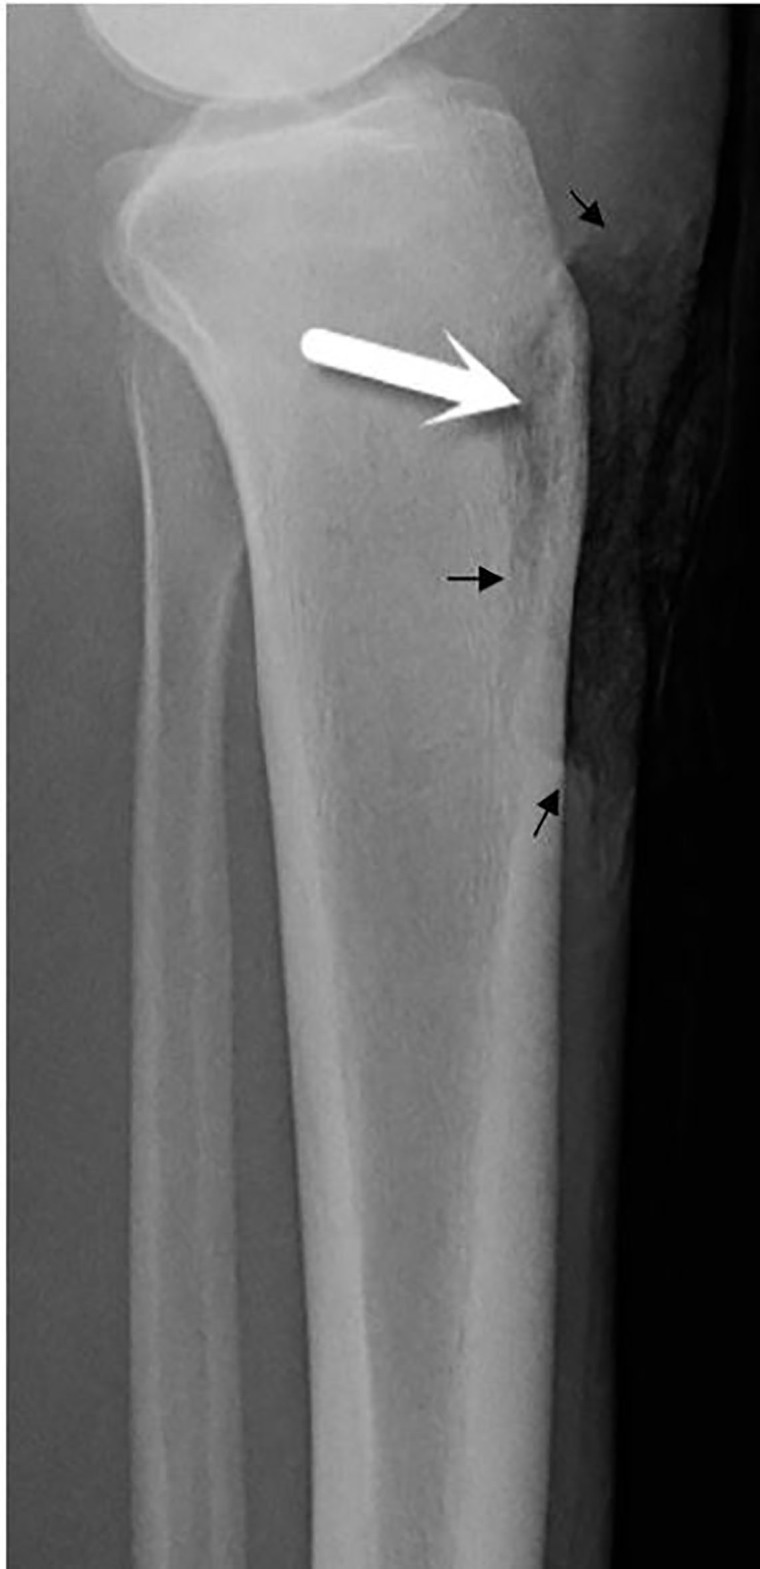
Lateral plain radiography demonstrating a soft tissue defect (black arrows) along the proximal anterior tibia with subtle cortical demineralization (white arrow).
